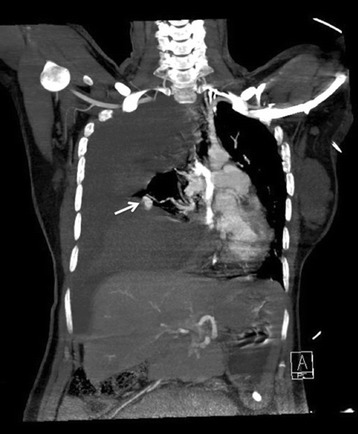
Coronal computed tomography image of the patient’s chest. A massive right-sided hemothorax and an arterial phase-enhanced lesion, compatible with a vascular anomaly (*white arrow*), in the lower lobe of the right lung
